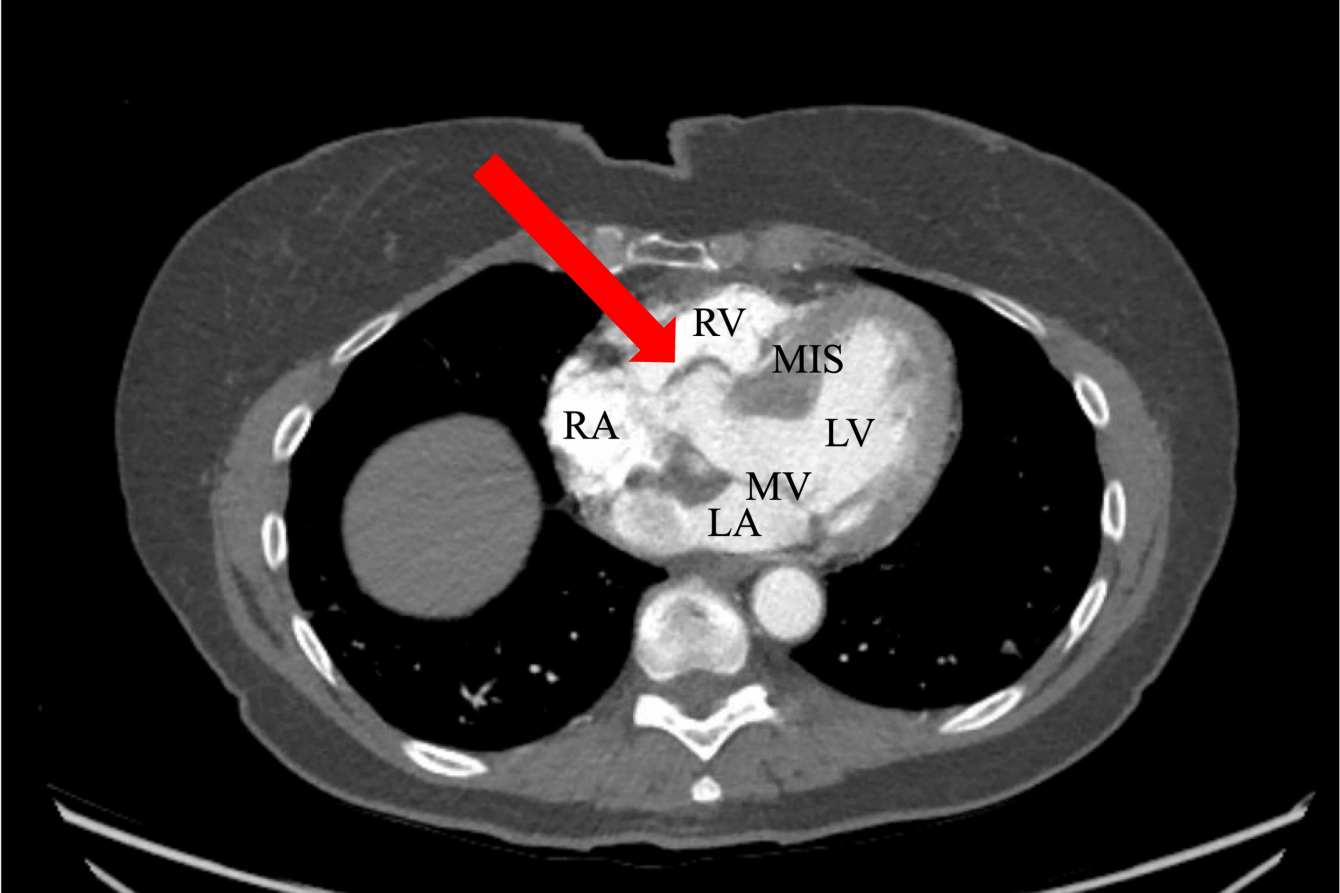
Computed tomography (CT) angiography of chest depicting the interventricular membranous septal aneurysm (arrow), right atrium (RA), right ventricle (RV), left atrium (LA), mitral valve (MV), left ventricle (LV), and muscular interventricular septum (MIS).
